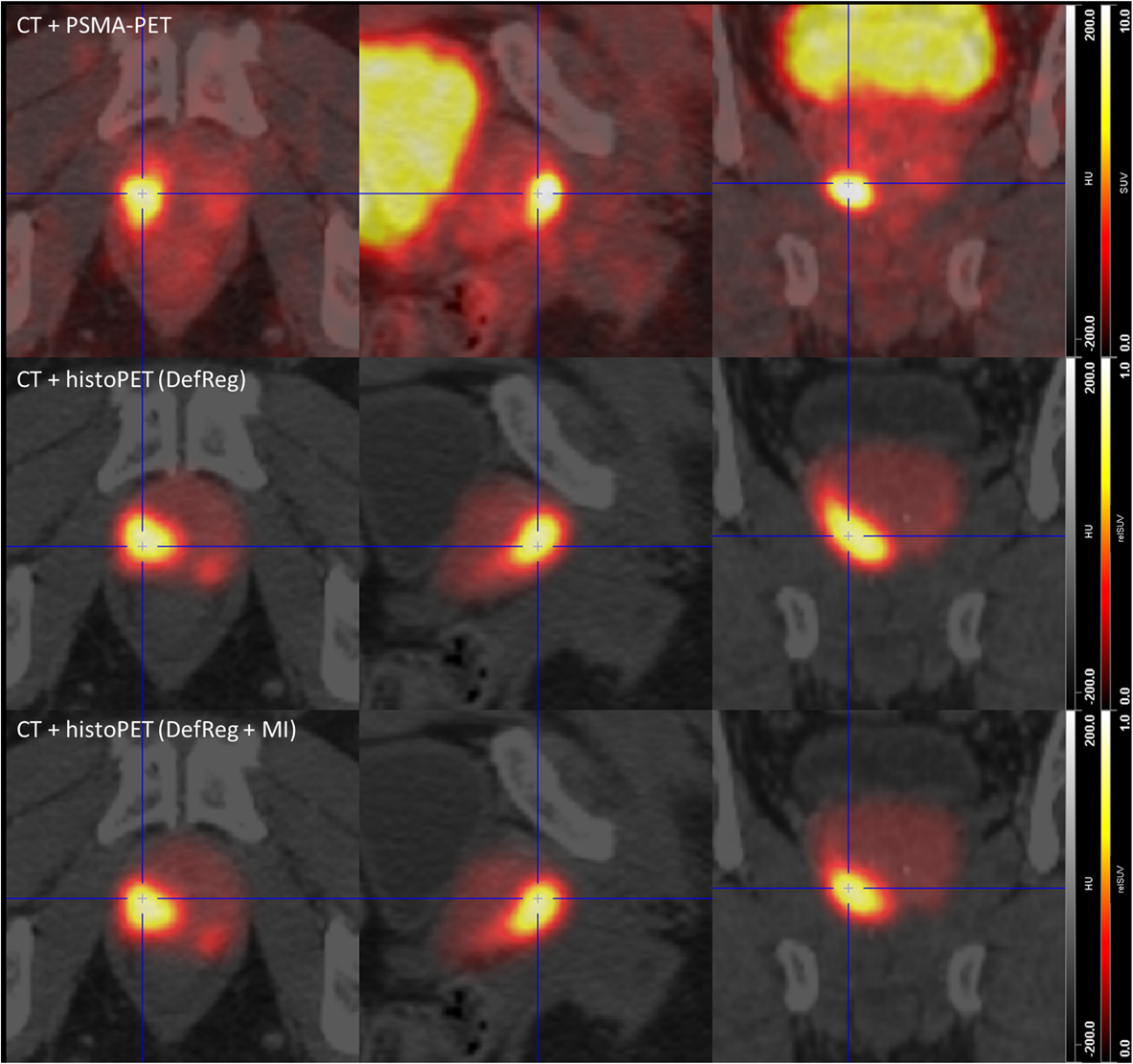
Visual comparison of PSMA-PET/CT and histoPET models according to DefReg and DefRegMI coregistration. The deformation of the 3D histoPET by the deformable registration algorithm is clearly visible. Results showed that DefReg is more sensitive to uncertainties in contours of the prostate on in vivo and ex vivo CT than the pure manual ManReg is. However, pattern agreement between PSMA-PET and histoPET with *R*
